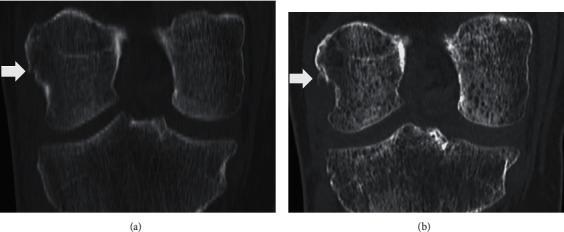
Patient case 2. Frontal plane CT assessment. (a) At day 1, showing the avulsed bony fragment of the femoral insertion of the LCL (white arrow). (b) At 4.5 months, showing bone union with mild calcification of the femoral attachment of the LCL (white arrow).
